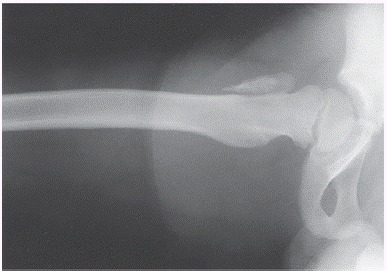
Radiography in frog position of the right hip, with anterior fracture dislocation or avulsion of the apophysis of the greater trochanter.
